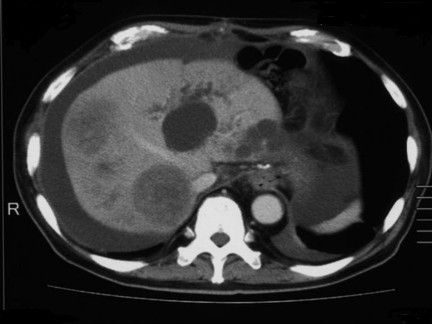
Abdominal computed tomography three months after surgery showed multiple metastatic lesions in the liver and ascites.
